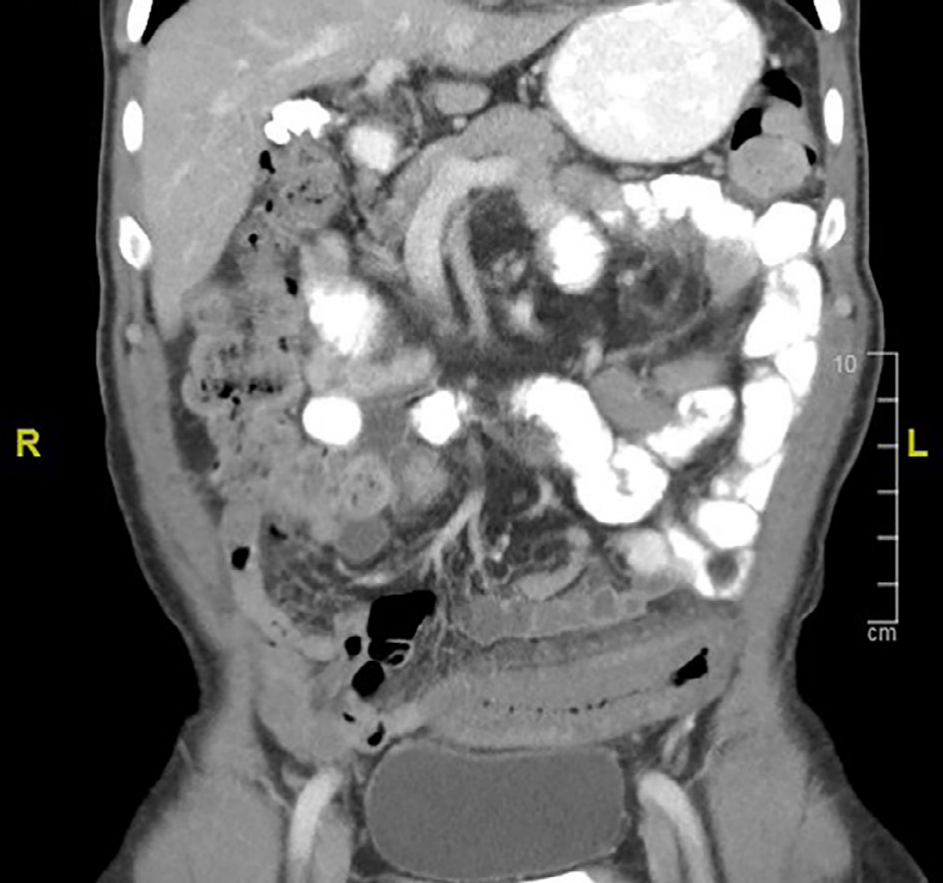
Computed topography (CT) abdomen (coronal view) showing contiguous concentric thickening of the distal descending colon to the rectum with stranding of the adjacent colonic fat.
